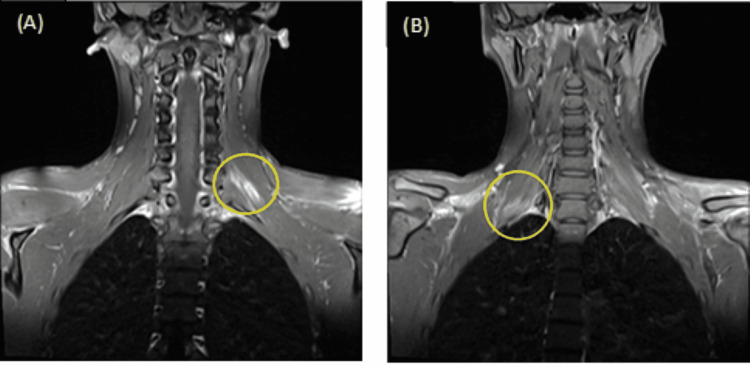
(A): MRI image showing swelling of the C6, C7, and C8 nerves and hematoma in the surrounding tissues of the left brachial plexus (inside the yellow circle), (B): MRI image showing thickening of the C6, C7 and C8 nerves of the right brachial plexus (inside the yellow circle)
